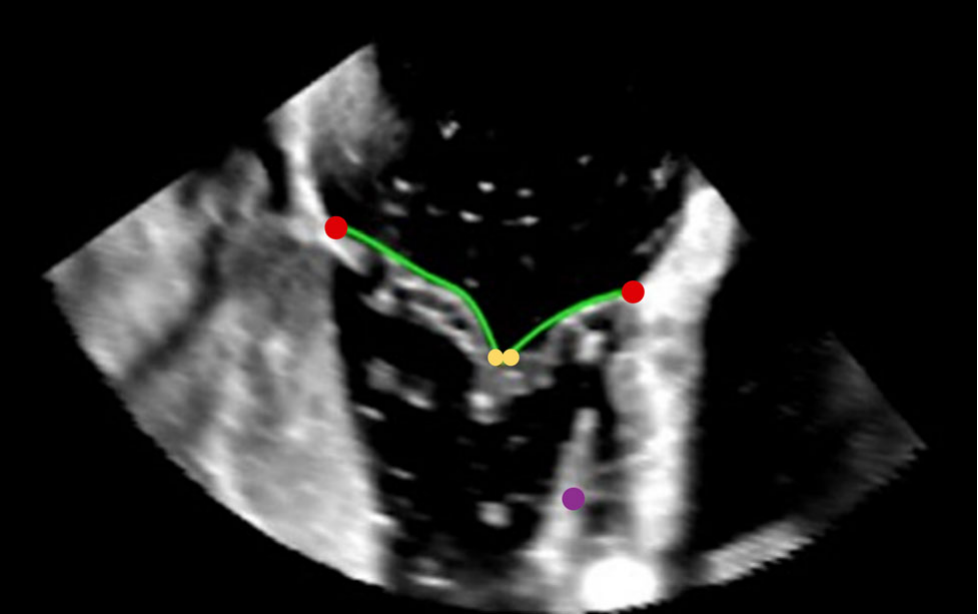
Echocardiographic imaging of the TV used for the segmentation: annulus (red points), leaflet profile (green lines), leaflet free margin (yellow dots) anterior papillary muscle (purple dot).
